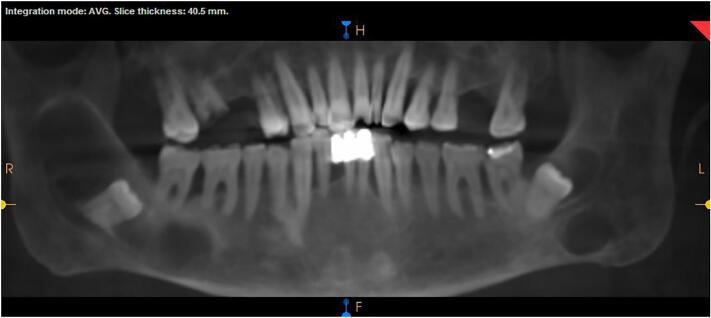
Reconstructed orthopantomograph from the patient's Cone Beam Computed Tomography showing three cyst-like lesions.
